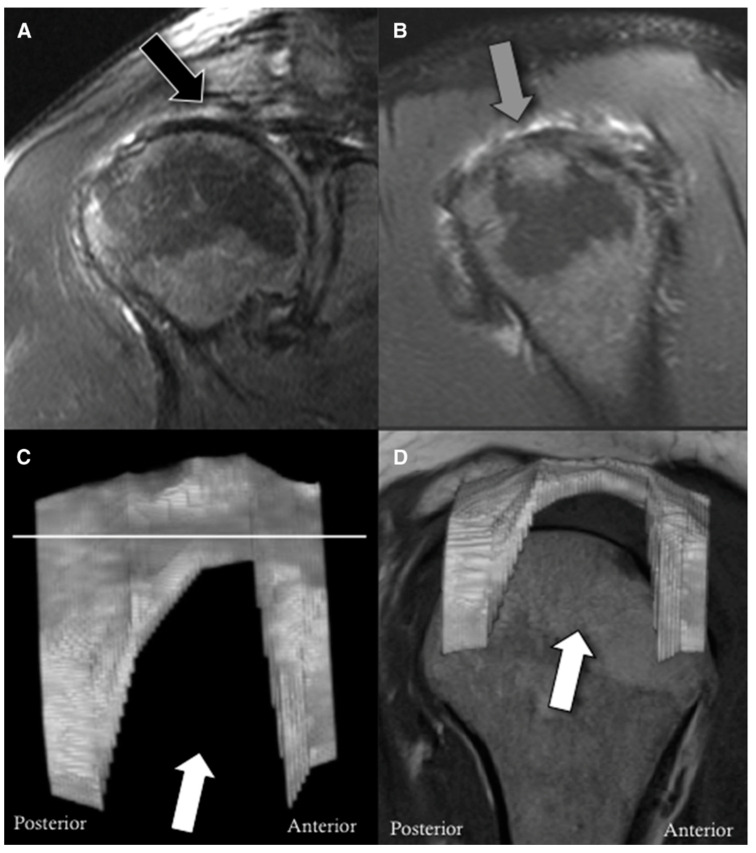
A 50-year-old man with a U-shaped rotator cuff tear. (A) Coronal and (B) sagittal fat-suppressed T2-weighted images of the right shoulder demonstrate a full-thickness tear of the supraspinatus tendon (black and grey arrow). (C,D) demonstrate 3D reconstructions of the supraspinatus and anterior infraspinatus tendons including a U-shaped tear (white arrows). The white line in C denotes the joint line [50]. (Reproduced from with permission from Ref. [50]. Copyright © 2023, published by Elsevier. All rights reserved.)
